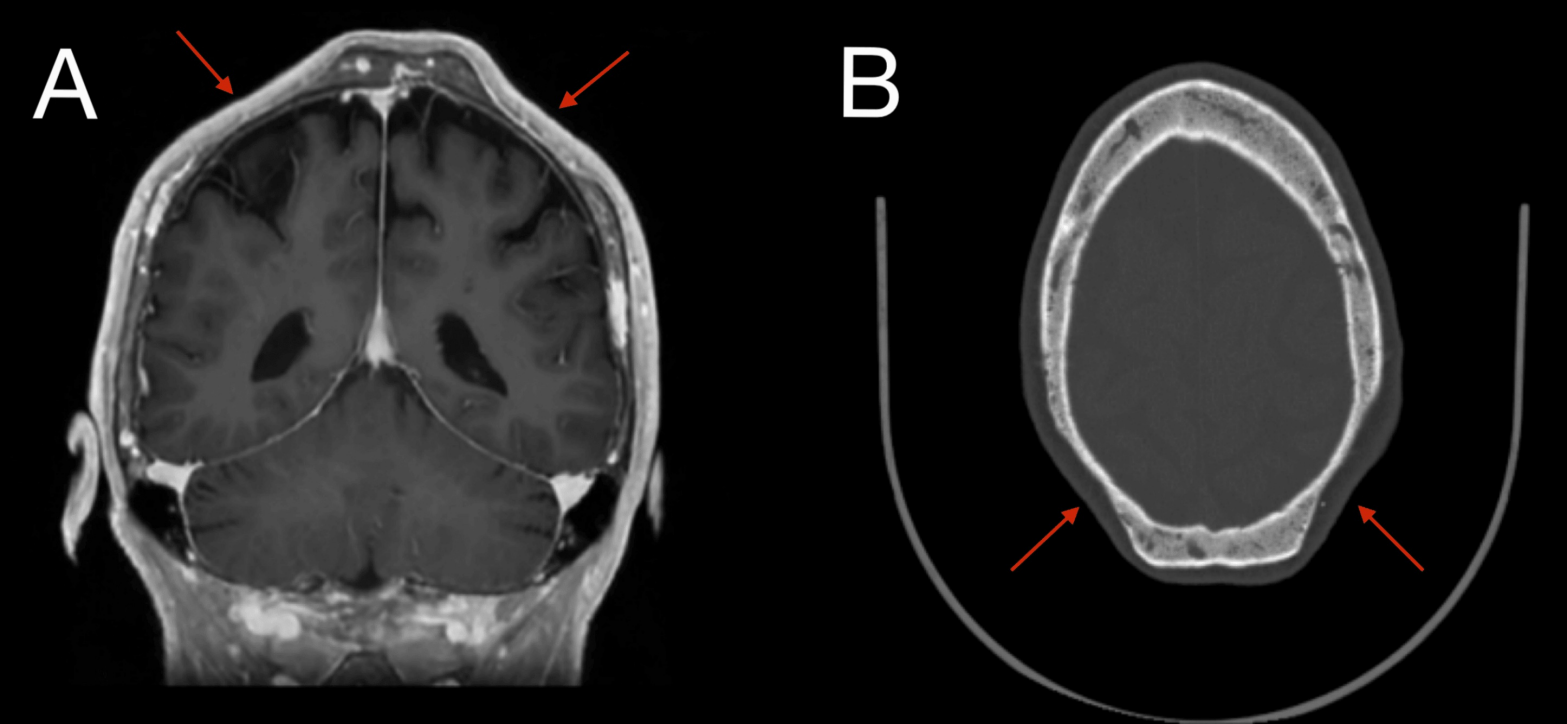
Biparietal osteodystrophy on MRI imaging. Coronal (A) and axial (B) views show bilateral thinning of the parietal bone and no underlying intracranial abnormalities. MRI: magnetic resonance imaging
